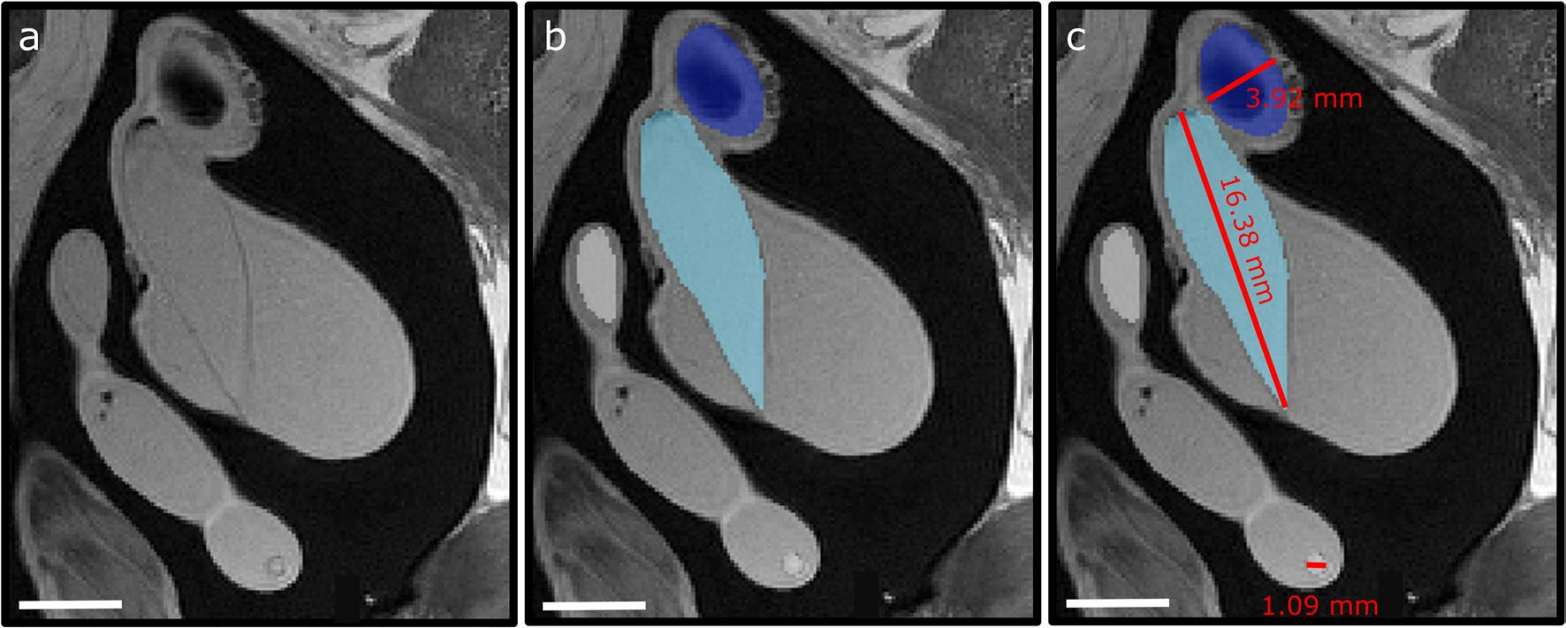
Linear measurement. An individual axial scan slice from the inner ear of a school shark (*Galeorhinus galeus*) showing (a) the original MR image, (b) the segmentation of inner ear structures, and (c) the linear measurement of the same inner ear structures. Dark blue = utricle; Light blue = saccule, Grey = semi-circular canals. Scale bar = 5 mm.
